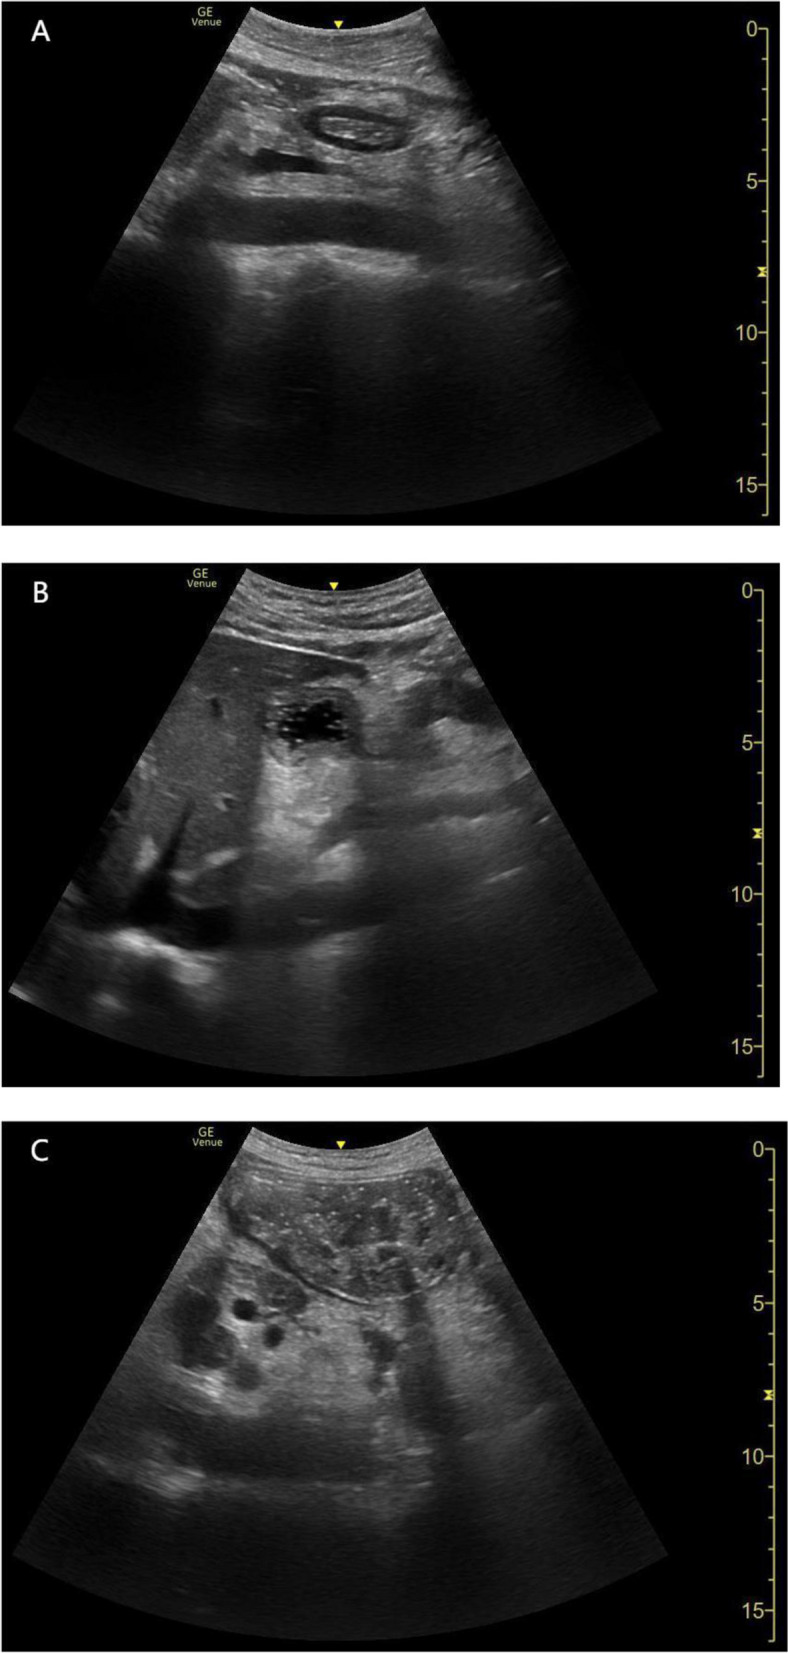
Sonographic images of the epigastric area with the gastric cancer patients in the right-lateral decubitus. Antrum: (a) empty; (b) fluid; (c) solid content
